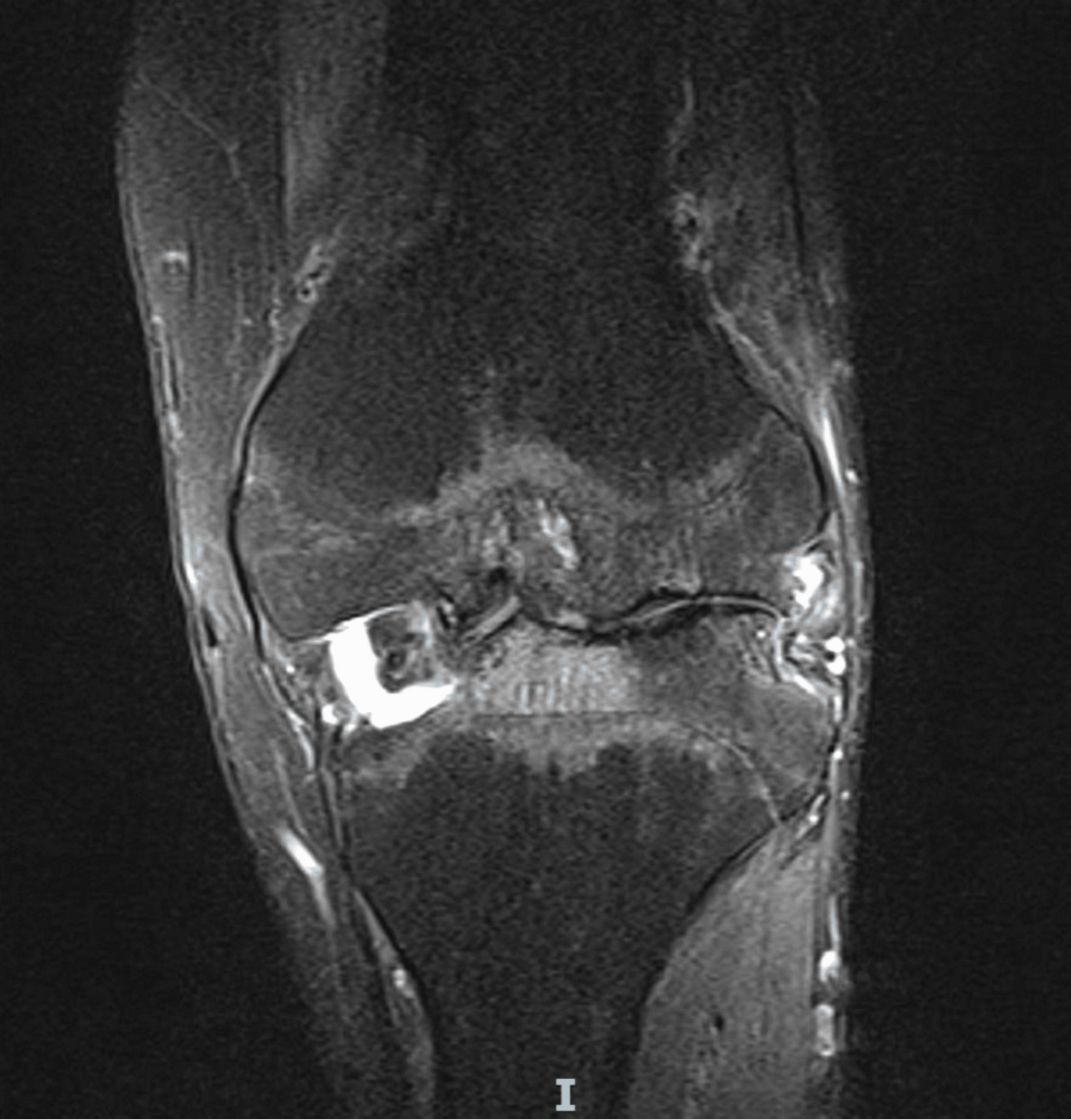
Preoperative coronal MRI of the left knee.
